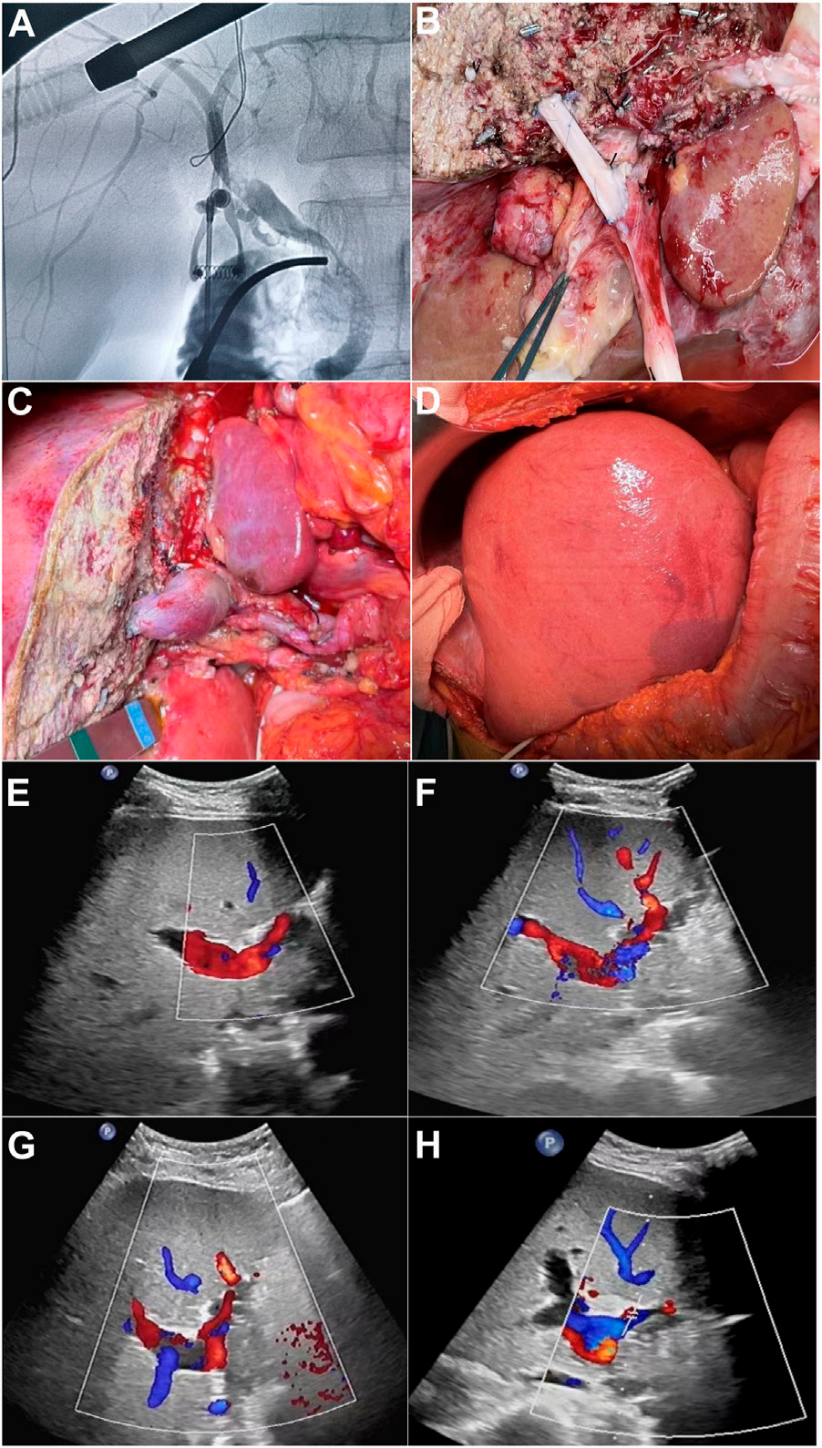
(A) Pre-splitting cholangiography of liver parenchyma. (B) Segment IV portal vein reconstruction before blood flow. (C) Segment IV portal vein reconstruction after blood flow. (D) After surgery/portal vein reconstruction, right three lobes. (E–H) Postoperative ultrasound images of segment IV portal vein bridging vessels of days 1, 3, 5, and 7, respectively.
